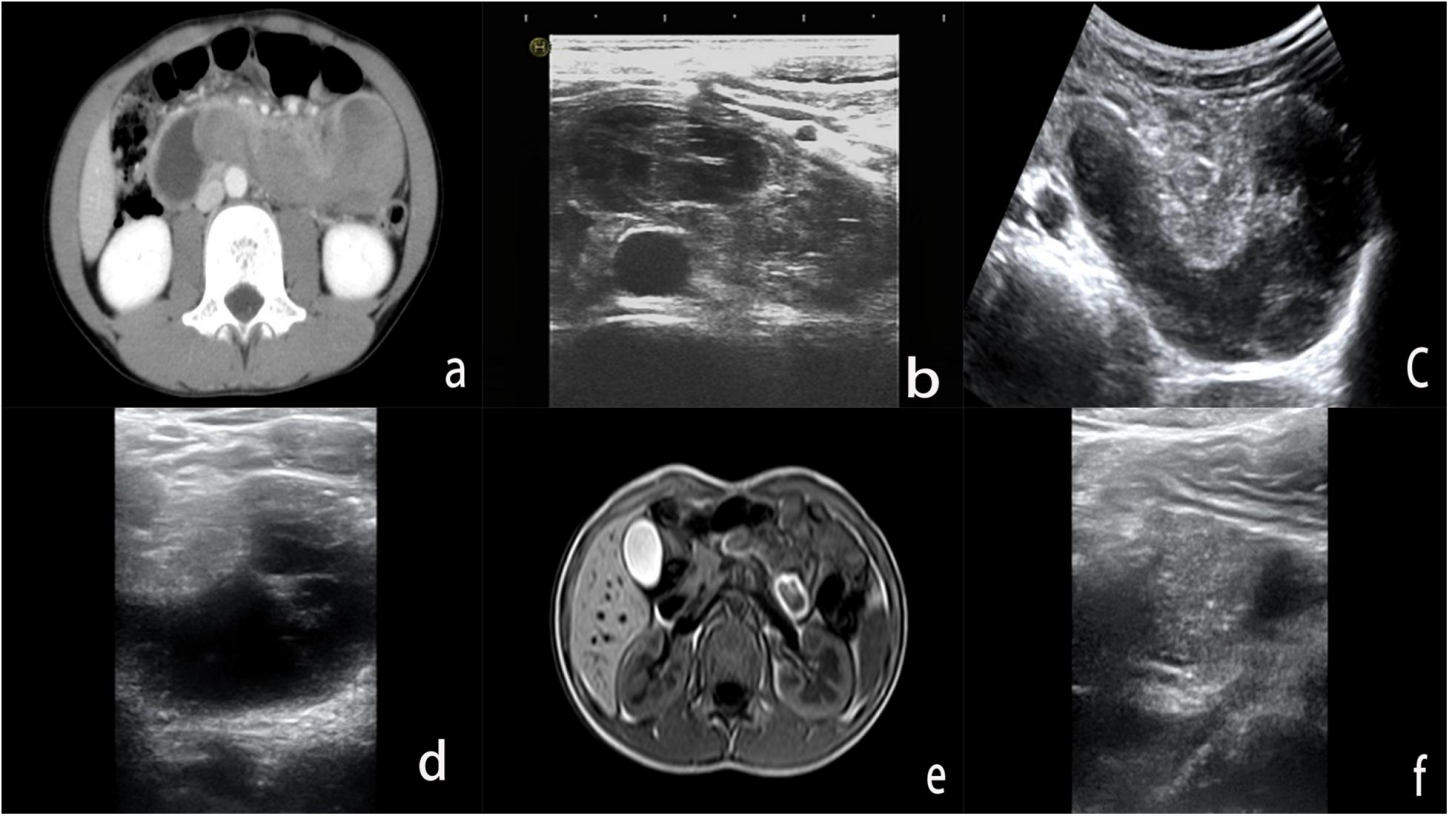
Imaging examination of duodenal wall hematoma in a child with car injury. (a) On the day of injury, CT scan showed a long, clustered, slightly high-density shadow in the horizontal and ascending intestinal walls of the duodenum, but no significant enhancement was observed after administration of contrast media. (b) High-frequency ultrasound shows the hematoma is located within the intestinal wall, between superior mesenteric artery and the abdominal aorta, presenting as a solid elongated elliptical heterogeneous echo. (c) On the 5th day of conservative treatment, abdominal ultrasound re-examination showed an enlarged hematoma in the shape of a sausage, with uneven internal echoes and partial cystic lesions. (d) On the 10th day of conservative treatment, high-frequency ultrasound showed a hematoma with a circular shape, mainly cystic inside, and abdominal artery compression. (e) On the 15th day of conservative treatment, magnetic resonance imaging showed a circular TI low signal surrounding high signal shadow in the duodenal wall, but no enhancement was observed. (f) On the 20th day of conservative treatment, high-frequency ultrasound showed solid hypoechogenicity in the horizontal part of the duodenal wall, with a significant reduction in volume compared to before.
